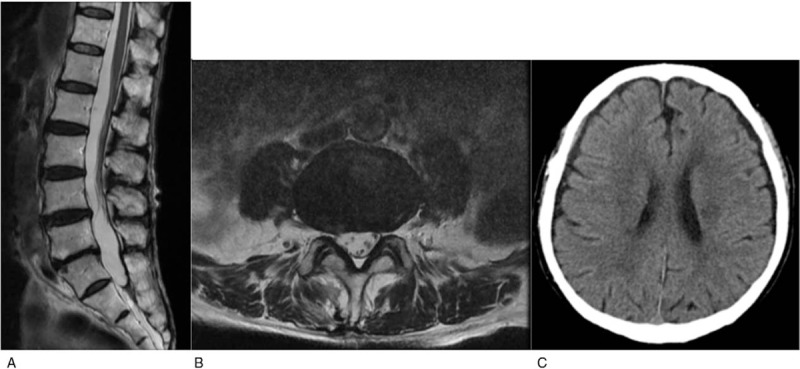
(A, B) Sagittal and axial T2-weighted spine images show complete resorption of the hematoma. (C) Non-contrast computed tomography shows significant resorption of the hematoma.
